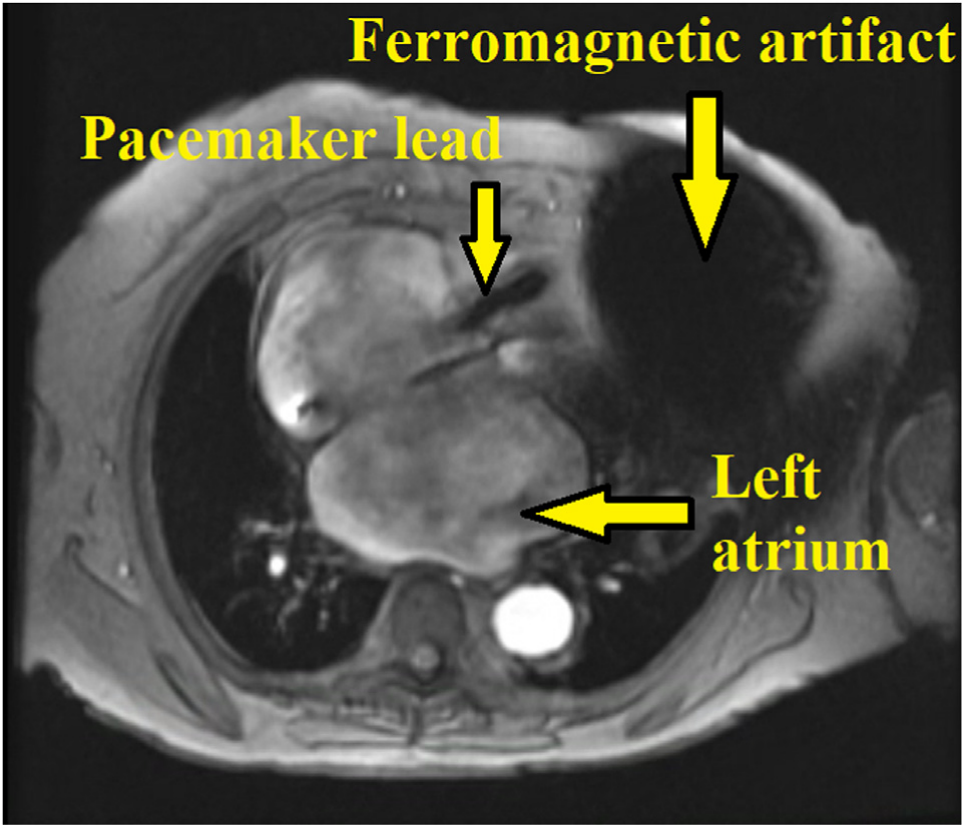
Cardiac magnetic resonance imaging (1.5 T scanner), cine spoiled gradient echo sequence, axial 4-chamber view, demonstrates the presence of severe ferromagnetic artifacts (*vertical arrows*) from biventricular pacemaker generator and leads that obscures visualization of the LV myocardium.
